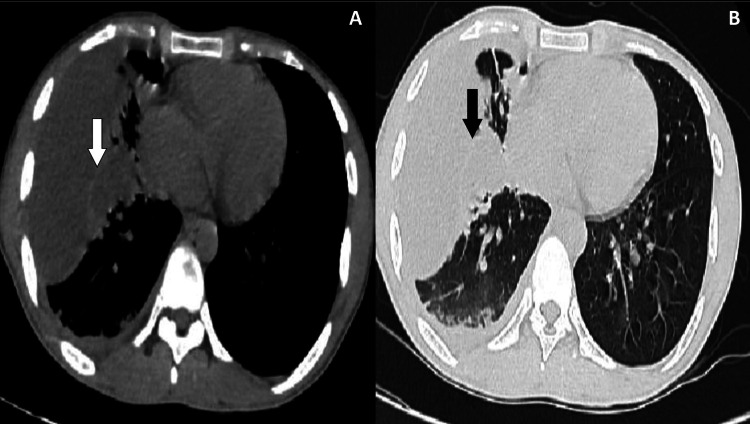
High-resolution computed tomography (HRCT) of the thorax showing loculated pleural effusion (A) Soft-tissue window: Axial HRCT image demonstrating a large right-sided pleural effusion with loculation. The white arrow indicates a loculated pleural fluid collection along the lateral aspect of the right hemithorax. (B) Lung window: Corresponding axial section showing loculated pleural effusion (black arrow) with underlying subsegmental collapse of the right lower lobe. The collapsed lung appears as increased parenchymal density adjacent to the effusion.
